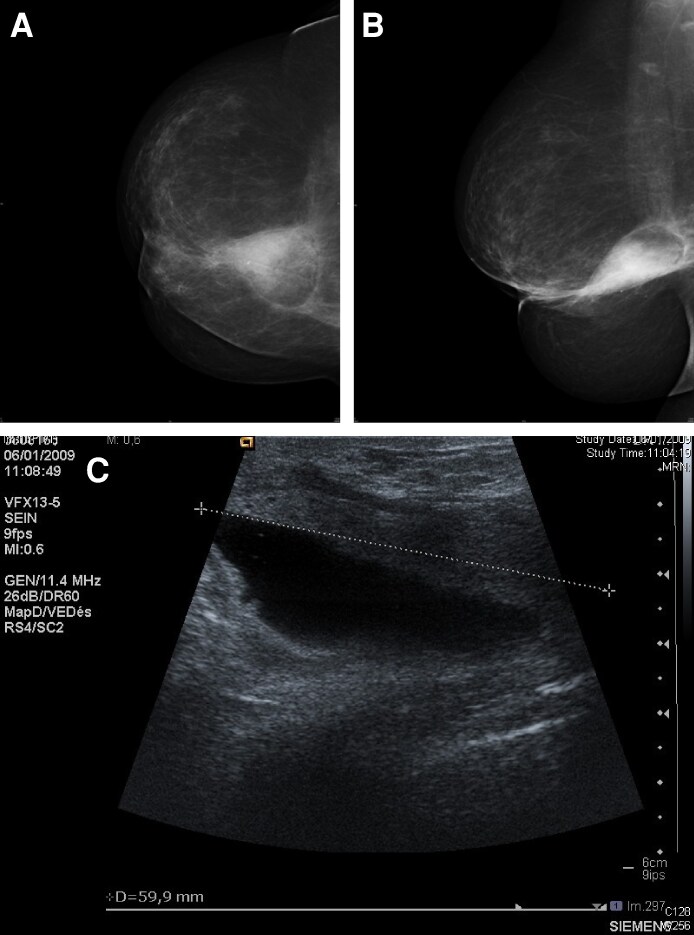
Imaging findings in a 60-year-old female with posttraumatic breast bisection following seat belt injury. (A, B) Mammography shows a well-defined radiolucent lesion with associated architectural distortion. (C) Ultrasound demonstrates a well-circumscribed anechoic cystic lesion with posterior acoustic enhancement, consistent with a typical fat necrosis (oil cyst).
